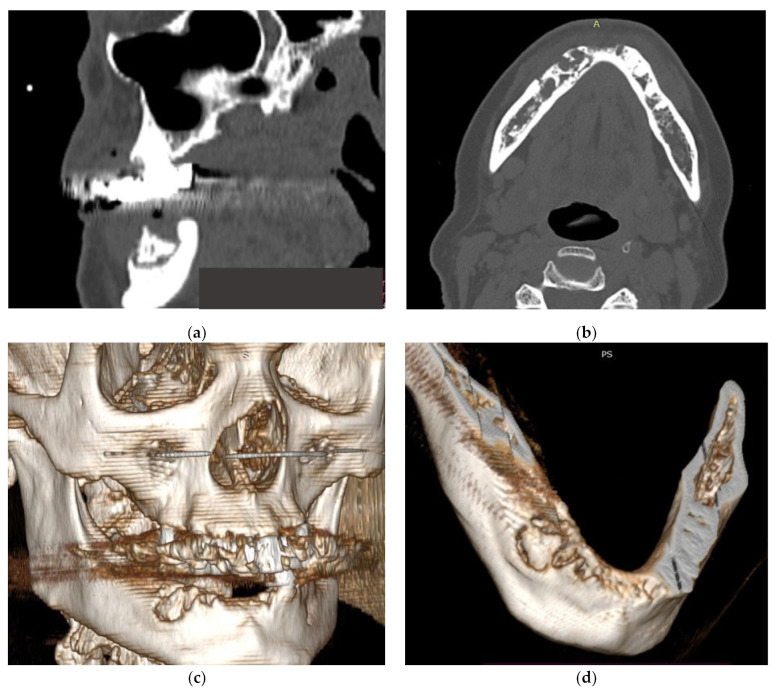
(a–d) CT aspects of patients with MRONJ.
